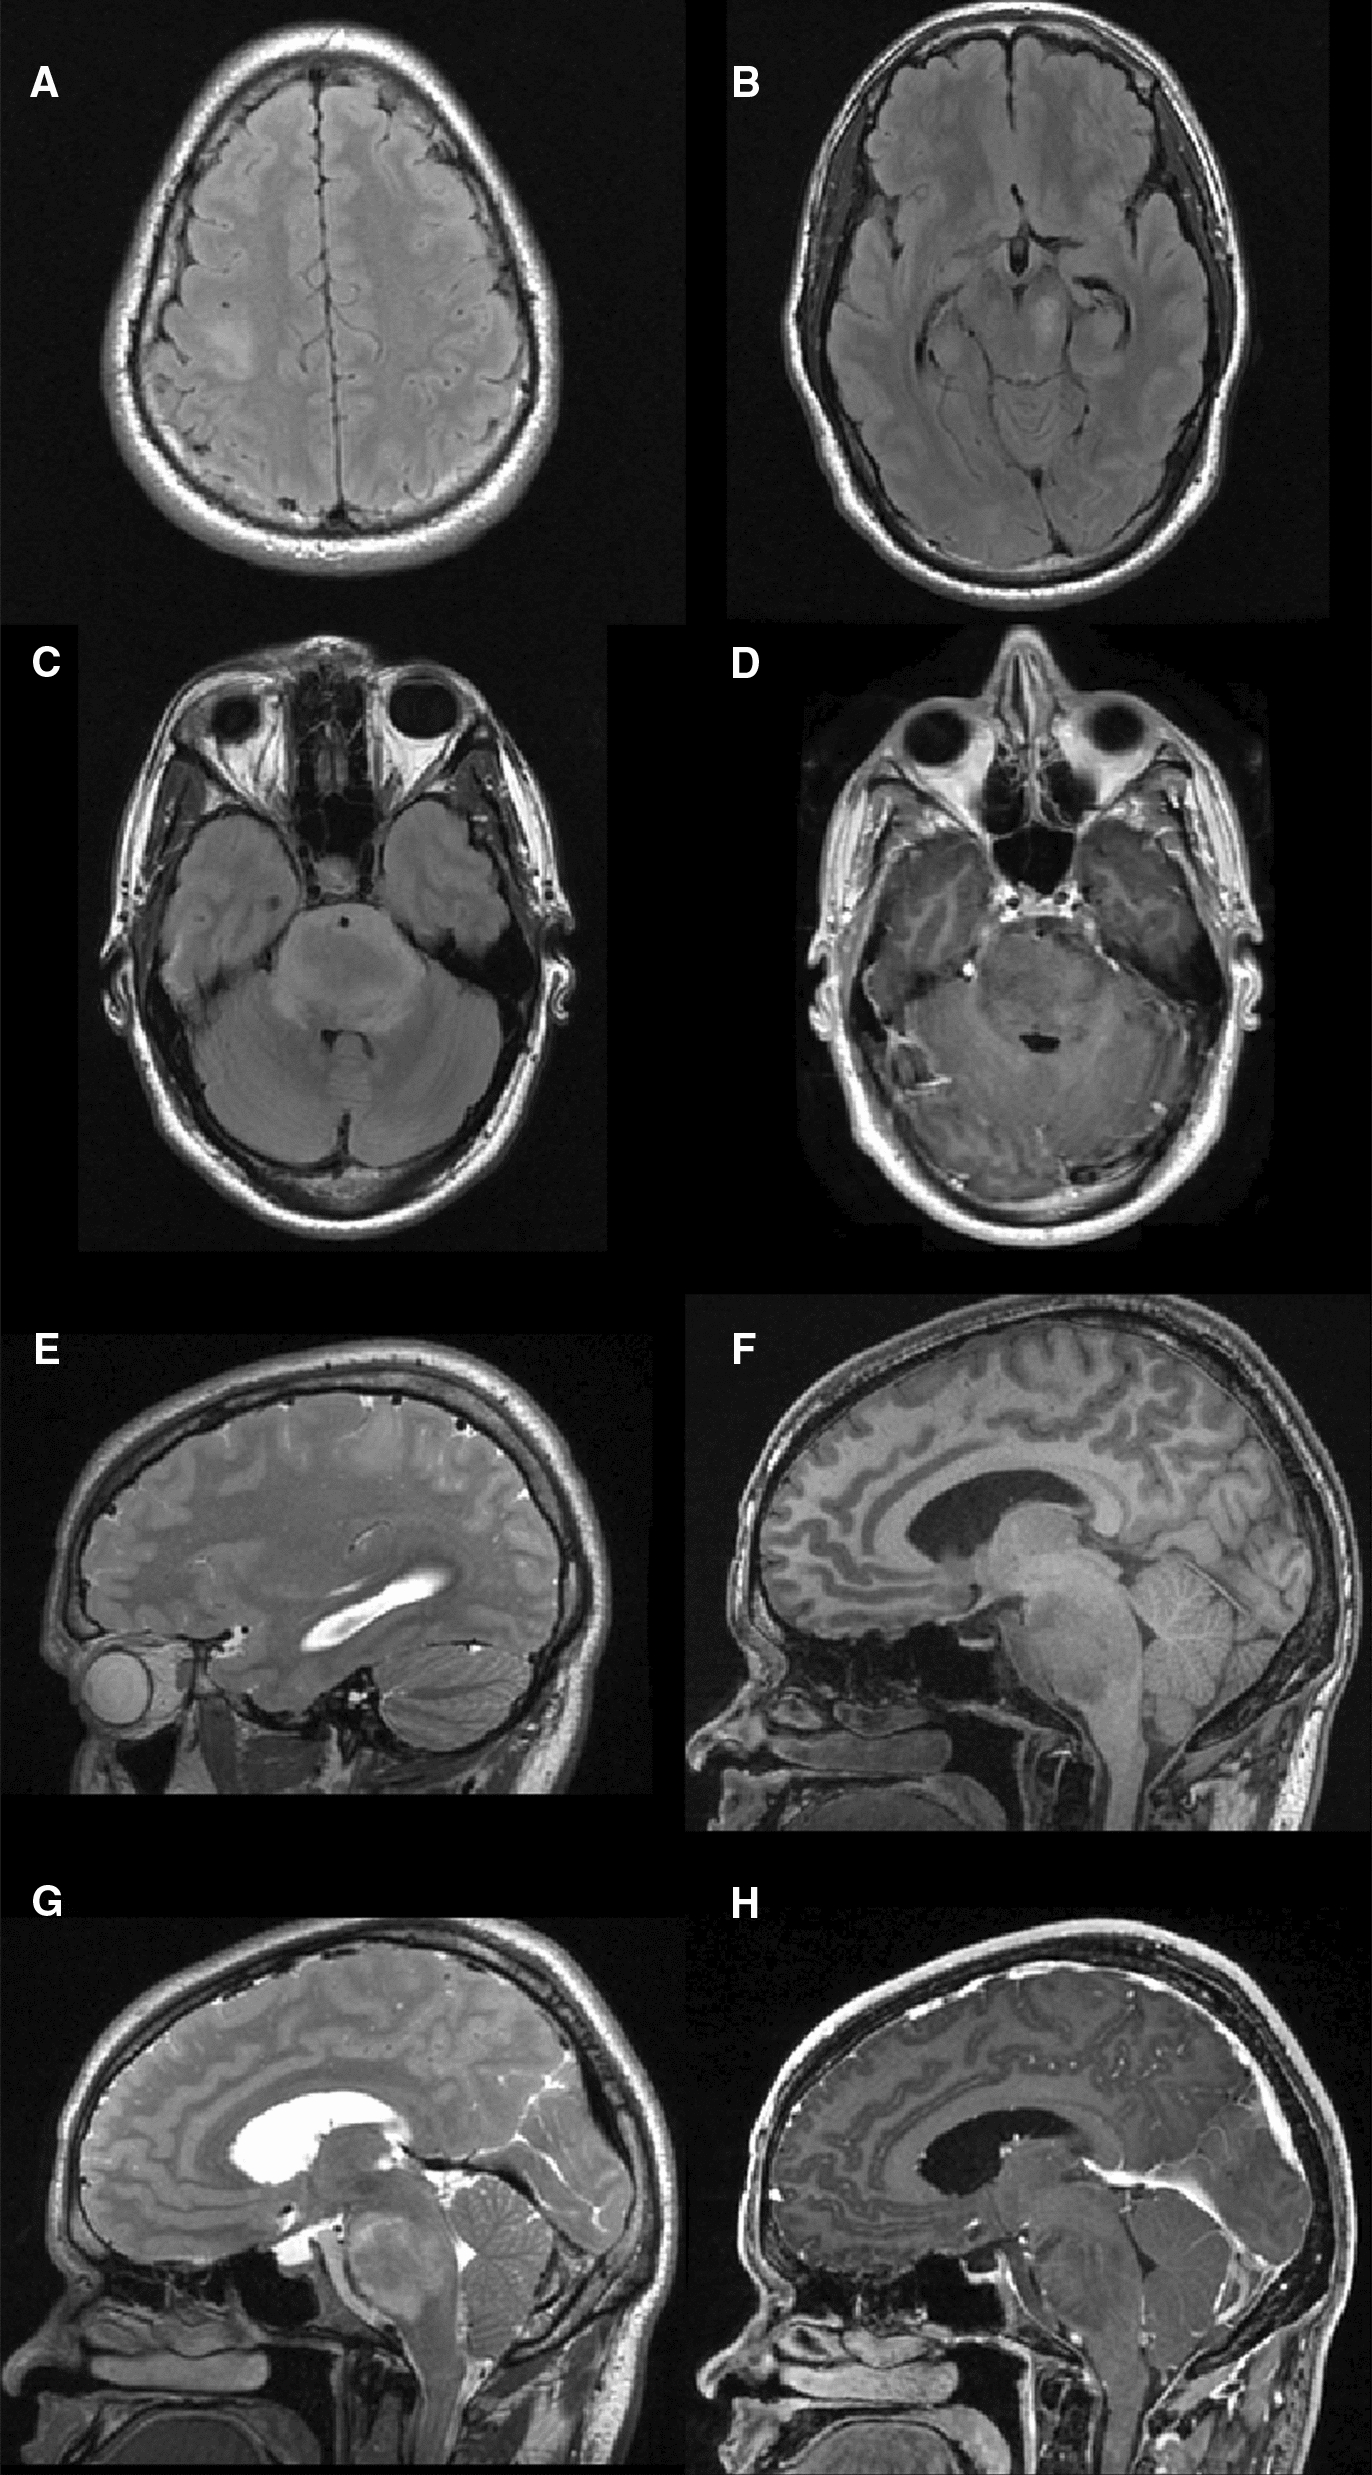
MRI of multifocal lesions on presentation. A Axial T2-FLAIR demonstrating a hyperintense round lesion, measuring 2.7 × 2.2 × 1.5 cm (CC × AP × transverse), in the precentral gyrus. Initial measurements prior to presentation at our institution: 2.2 × 1.7 × 1.5 cm. B Left greater than right hyperintensity in the cerebral peduncles on axial T2-FLAIR. C Axial T2-FLAIR demonstrates a heterogenous, hyperintense lesion in the pons, measuring 3.9 × 3.1 × 4.4 cm, with extension into bilateral cerebellopontine cisterns and middle cerebellar peduncles. Encasement of the basilar artery is also present with no obstruction of flow. Initial measurements prior to presentation at our institution: 3.5 × 3.1 × 4.3 cm. D Axial T1 post-contrast image of the pontine lesion demonstrating subtle patchy contrast enhancement. E Sagittal T2 image of the precentral lesion with compression of the central sulcus. F Large heterogenous, T1 hypointense pontine lesion extending from the floor the fourth ventricle to the prepontine cistern ventrally. G Pontine lesion as described with heterogenous hyperintensity on this sagittal T2 slice. H Subtle patchy enhancement is again appreciated within the pontine lesion on this T1 post-contrast sagittal slice
